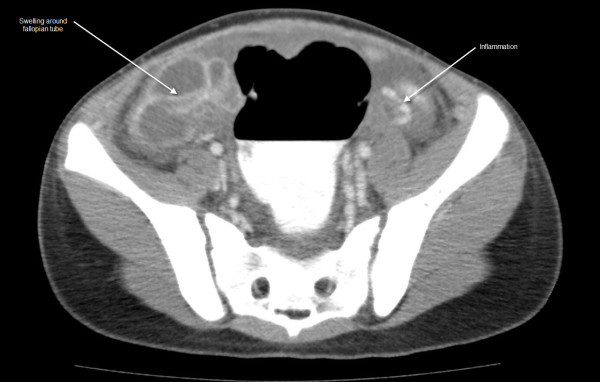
CT scan of the abdomen and pelvis with intravenous and oral contrast. Swelling and inflammation are shown around the fallopian tube.
